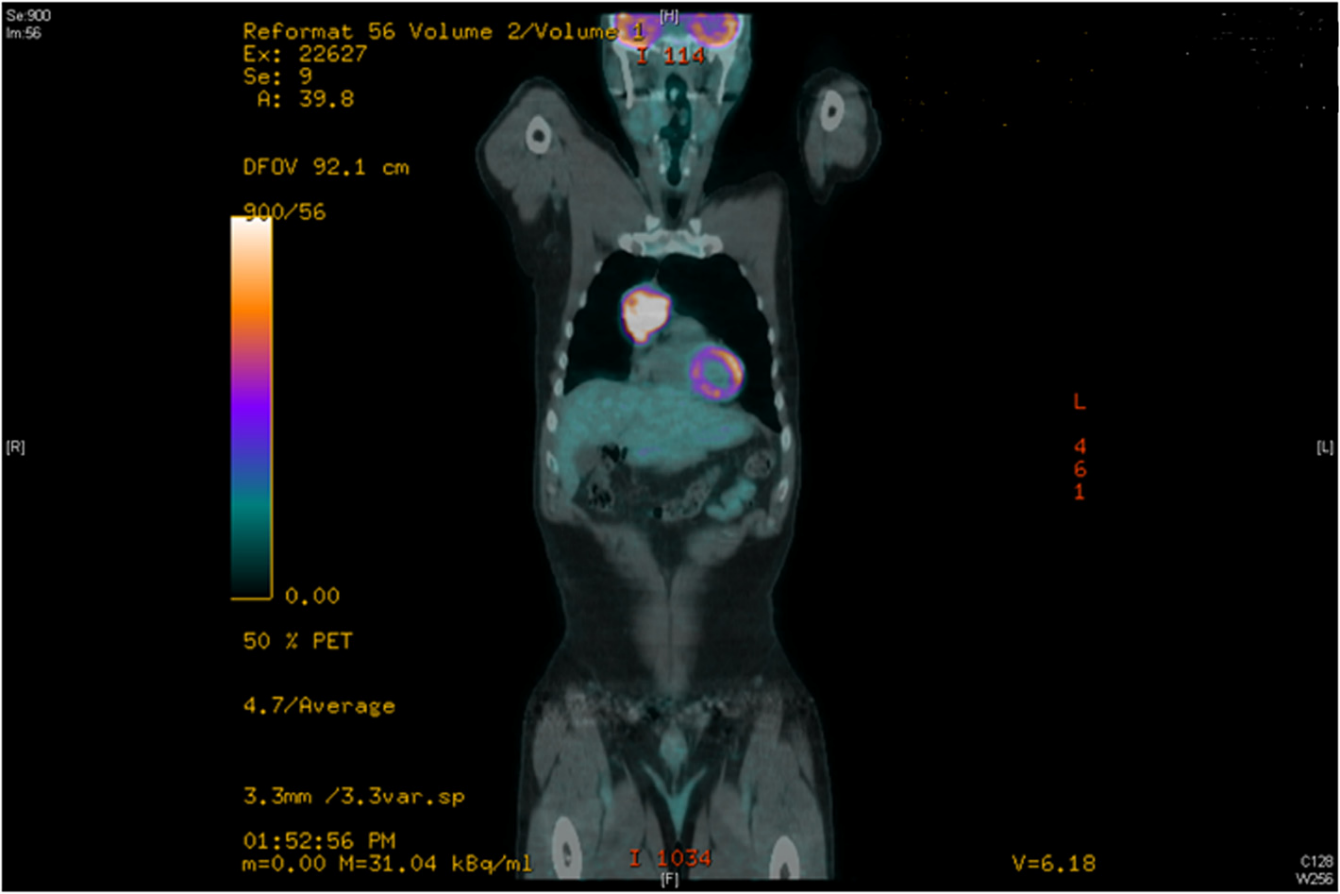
Positron emission tomography (PET) CT with intensely hypermetabolic 5.2 × 3.8 cm homogeneous anterior mediastinal mass (SUV max 15.94).
